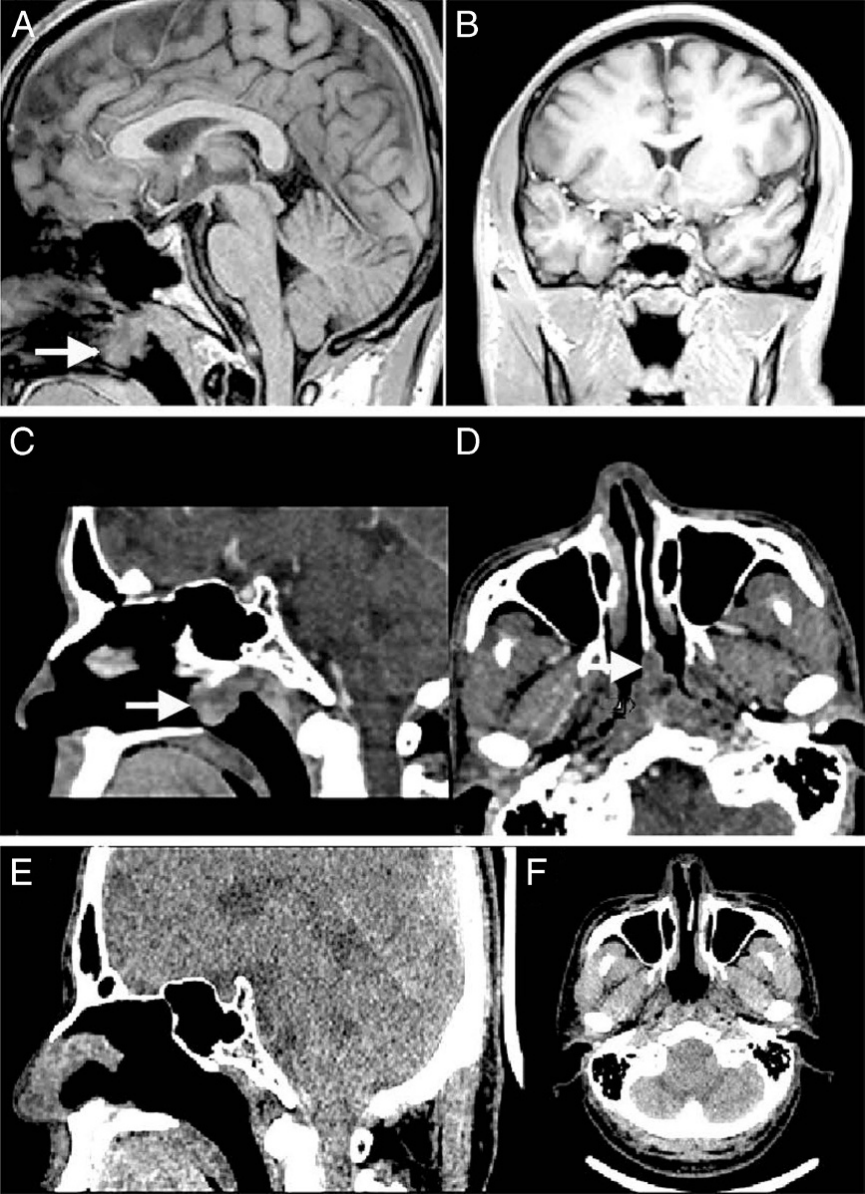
MRI and CT images. A &B, MRI of the pituitary showing a normal pituitary gland and an ectopic pituitary tumor in the nasopharynx (white arrow). C &D, CT scan showing a 1.9 × 1.7 cm mass in the nasopharyngeal cavity (white arrows). E &F, CT scan 48 months post surgery.
